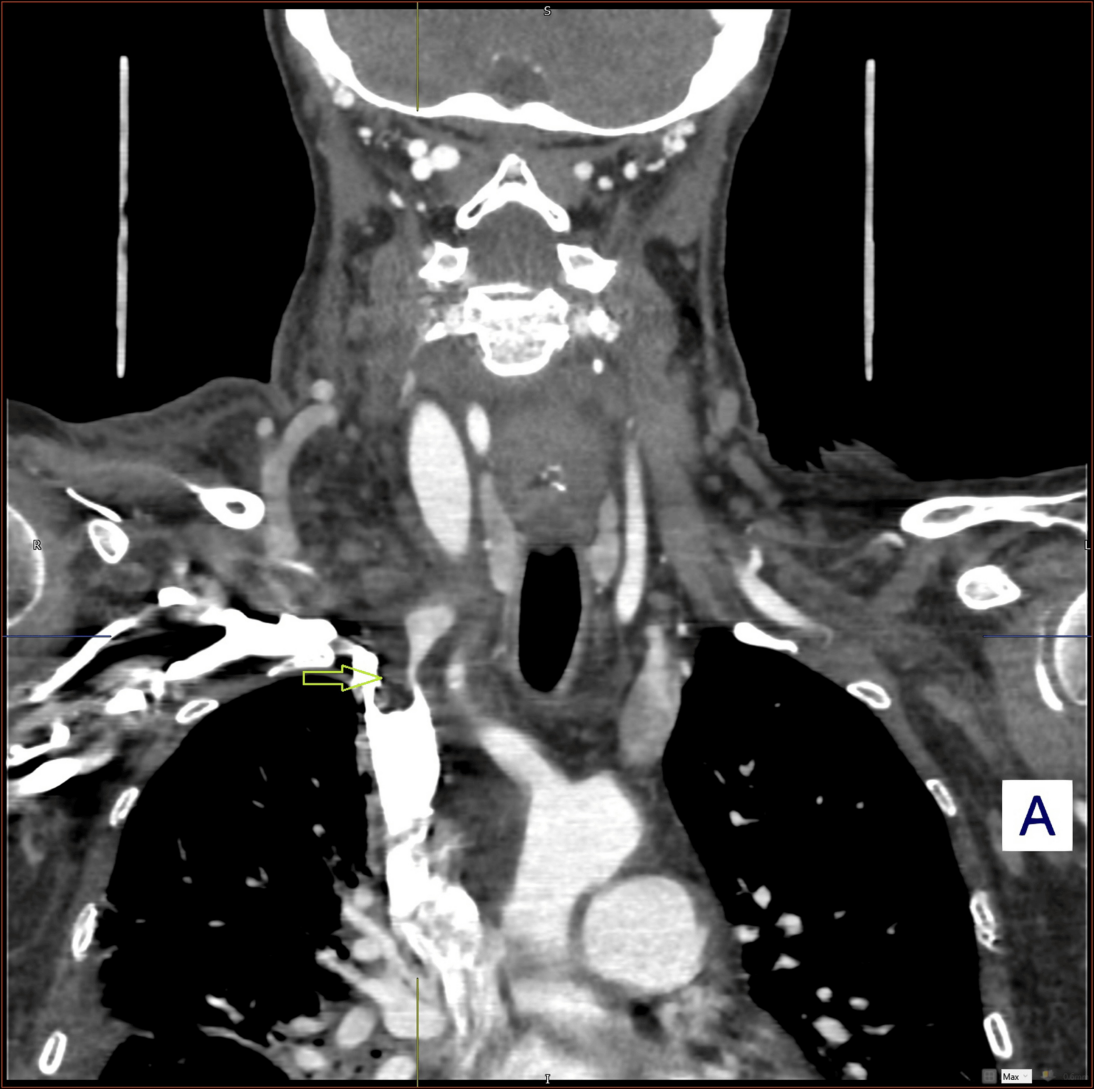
Coronal CT angiography scan showing thrombus in the right internal jugular vein (arrow).
